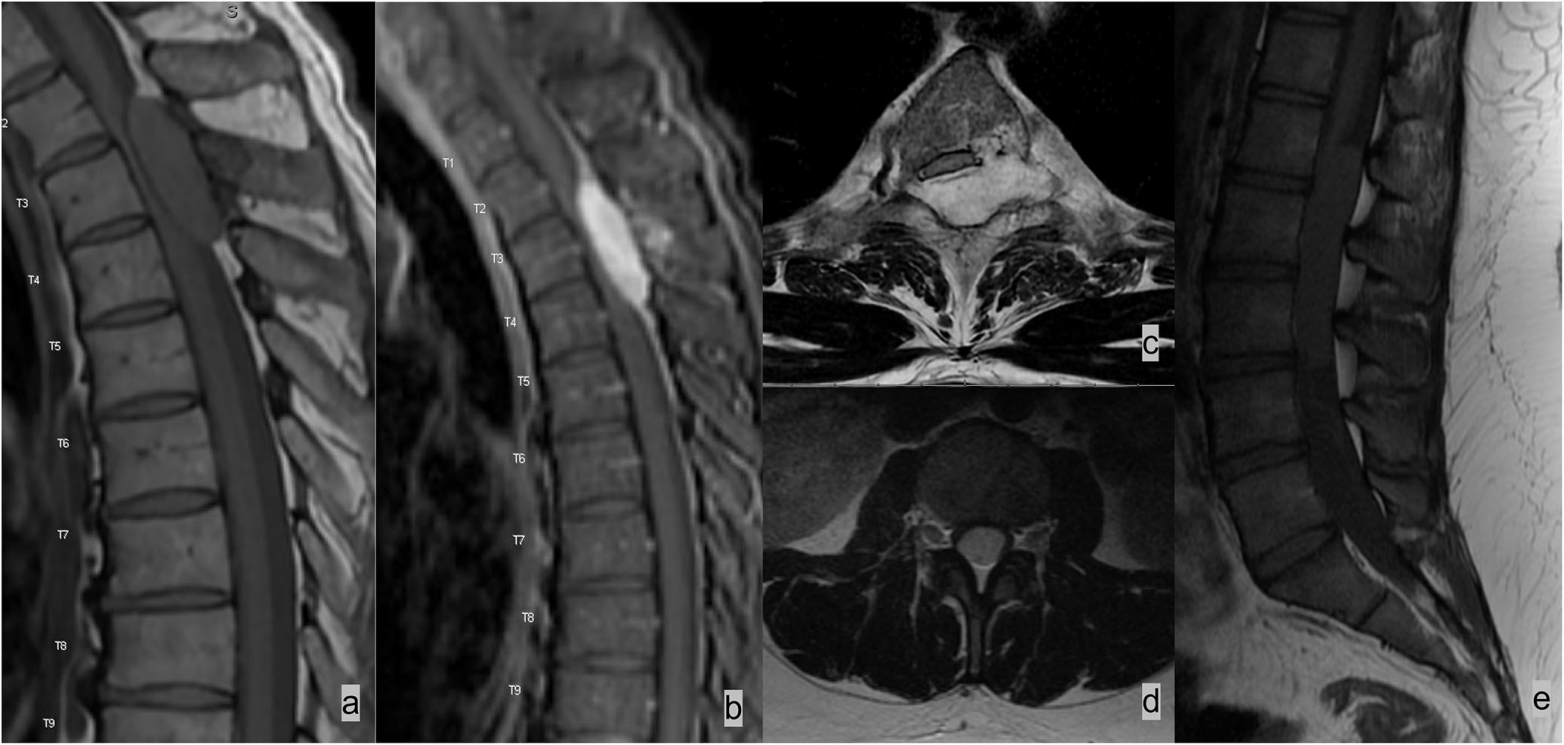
Two different patients with (a–c) epidural and (d,e) intradural spinal masses. (a–c) Patient 1 had spinal epidural hemangioma. MRI demonstrated a T2 hyperintense enhancing mass in the left dorsal and lateral epidural spaces compressing and displacing the spinal cord. Lesions in the epidural space results in effacement of the epidural fat and inward displacement of T1 and T2 hypointense thecal sac. (c,d) Patient 2 had myxopapillary ependymoma, an intradural mass, which expands the dural sac and compresses cauda equina nerve bundles. The epidural fat is preserved in an intradural lesion.
